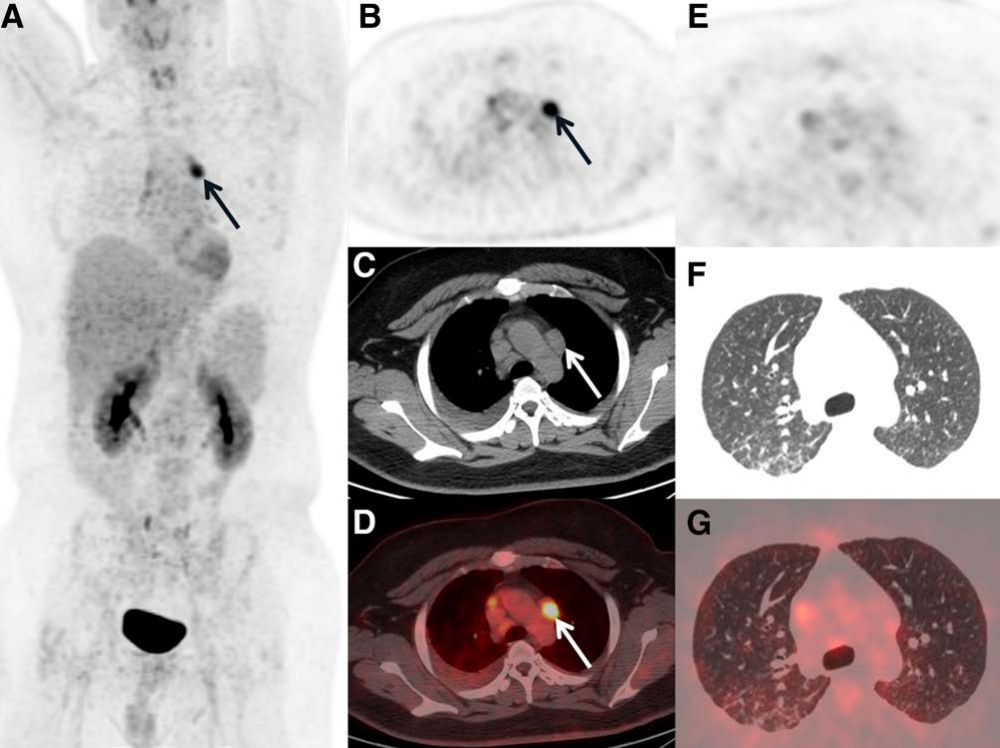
Only a few hypermetabolic lymph nodes and nodules remained existing in the mediastinum (arrows, A: MIP, B: PET, C: CT, D: fused PET/CT) and lung (E: PET, F: CT, G: fused PET/CT) after two months anticryptococcal therapy. Besides the metabolism of spleen back to normal. CT = computed tomography, MIP = Maximal Intensity Projection, PET = Positron Emission Tomography.
